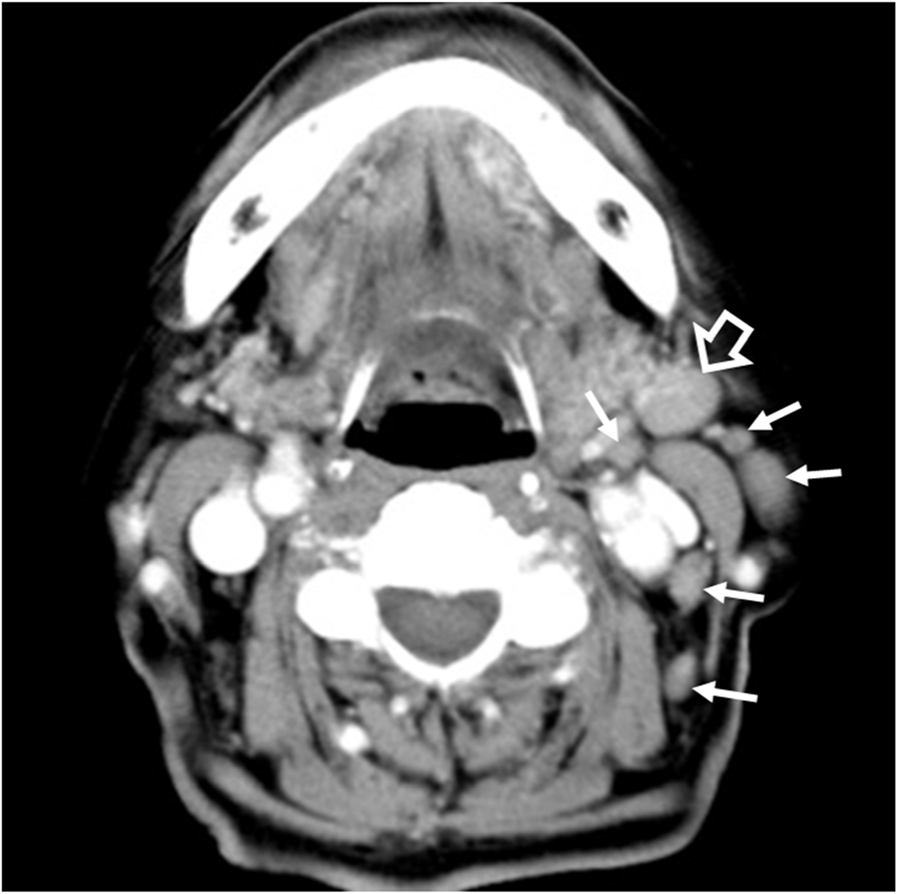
Computed tomography of a 77-year-old woman with left parotid MALToma and nodal disease. Axial contrast-enhanced image shows a slightly enlarged reactive appearing node (open arrow) and a cluster of small non-enlarged nodes (arrows) in the left upper internal jugular chain
